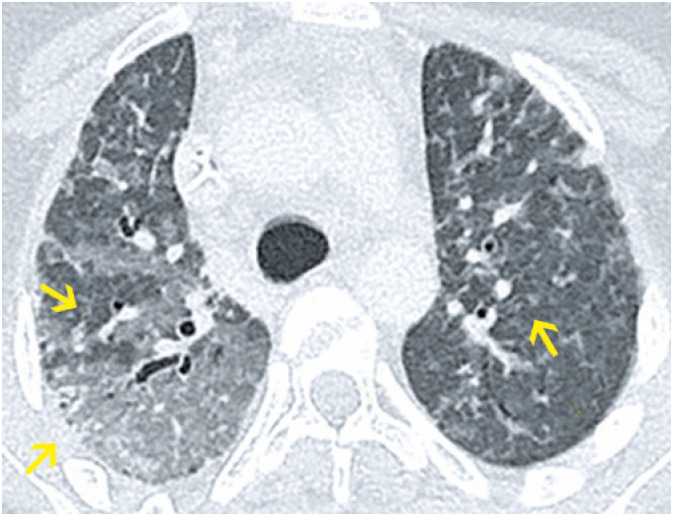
A 15-year-old female patient presented with sudden dyspnea. Personal medical history: immunosuppression. Computed tomography (axial): ground-glass opacities and bilateral peribronchovascular micronodules, accompanied by small foci of irregular consolidation. Diagnosis confirmed by bronchoalveolar lavage
